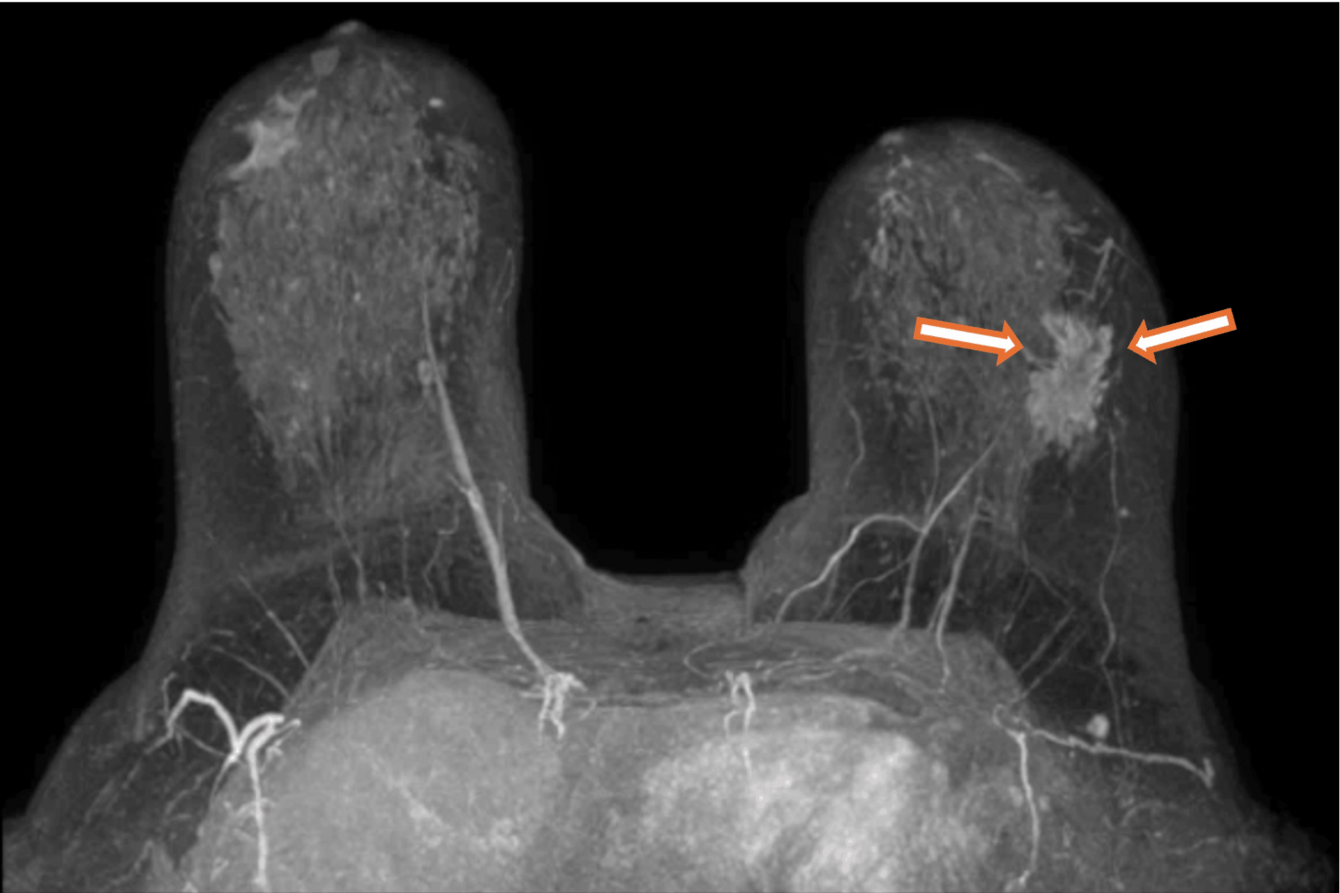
Breast MRI after neoadjuvant chemotherapy The two arrows indicate the residual tumor in the outer quadrants of the left breast, showing partial response to treatment MRI: magnetic resonance imaging
